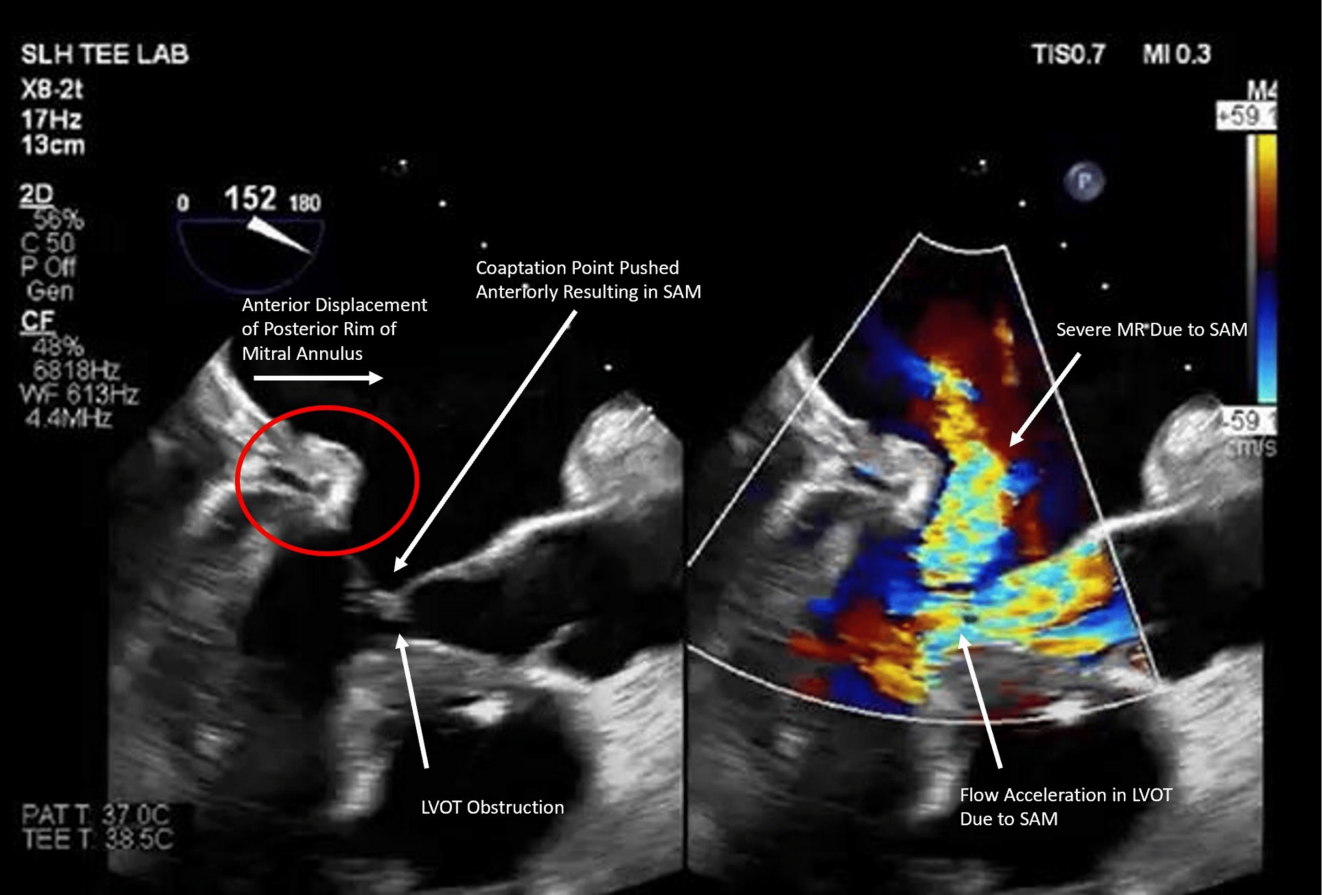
Transesophageal echocardiogram. Transesophageal echocardiogram with color Doppler (right) showing severe MR secondary to valvular SAM post-heart transplant. The posterior mitral annulus is anteriorly displaced secondary to reflected tissue (red circle), causing the coaptation point to be similarly displaced anteriorly and the development of LVOT obstruction. SAM, systolic anterior motion; LVOT, left ventricular outflow tract; MR, mitral regurgitation
